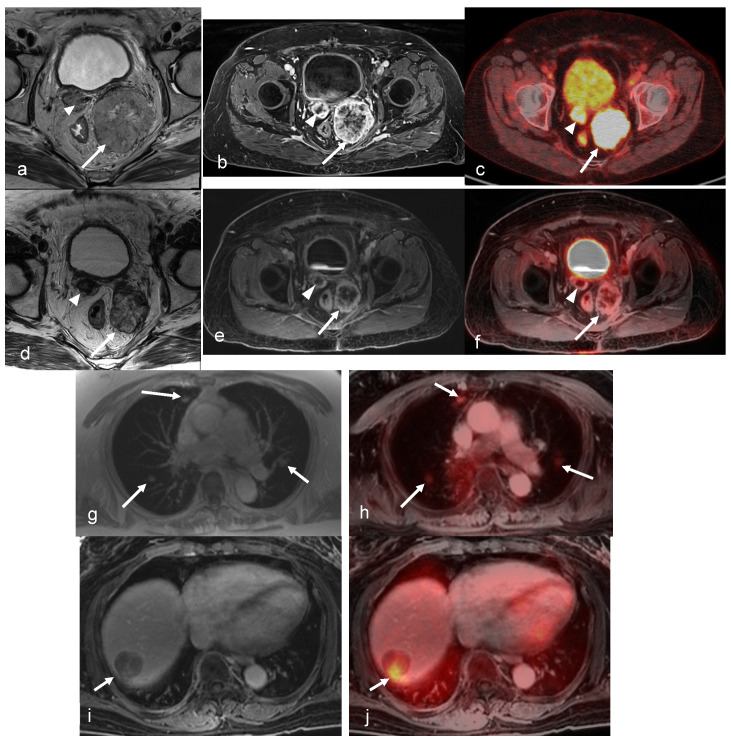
A 68-year-old female’s history of stage IVb endometrioid endometrial cancer who underwent systemic therapy, hysterectomy, and bilateral salpingo-oophorectomy with recurrent disease and pelvic implants in the vaginal cuff. Axial oblique small FOV T2-weighted (a) and axial post-contrast T1-weighted fat-saturated images (b) show two T2 intermediate signals, heterogeneously enhancing pelvic implants in the vaginal cuff (arrow and arrowhead). Fused PET/CT (c) images show intense FDG uptake in the pelvic implants (arrow and arrowhead). After external beam radiation therapy and brachytherapy, axial oblique small FOV T2-weighted (d) and axial post contrast T1-weighted fat-saturated images (e) show a decrease in size and the enhancement of the two pelvic implants in the vaginal cuff (arrow and arrowhead). Fused PET/MRI images (f) show a decrease in FDG uptake in the pelvic implants (arrow and arrowhead) with the small residual rim of a viable tumor, compatible with the partial local treatment response. (g) The axial in-phase image of the chest shows three new pulmonary nodules, most likely metastasis (arrowheads). A fused PET/MRI image (h) shows FDG uptake in the pulmonary nodules (arrowheads). (i) An axial post-contrast T1-weighted fat-saturated image shows a new heterogeneously enhancing metastatic liver mass with focal intense FDG uptake on fused PET/MRI images (arrowhead in (j)). (Courtesy of Vipul Sheth, MD, PhD and Negaur Iranpour, MD, Department of Radiology, Stanford University, Stanford, CA, USA).
